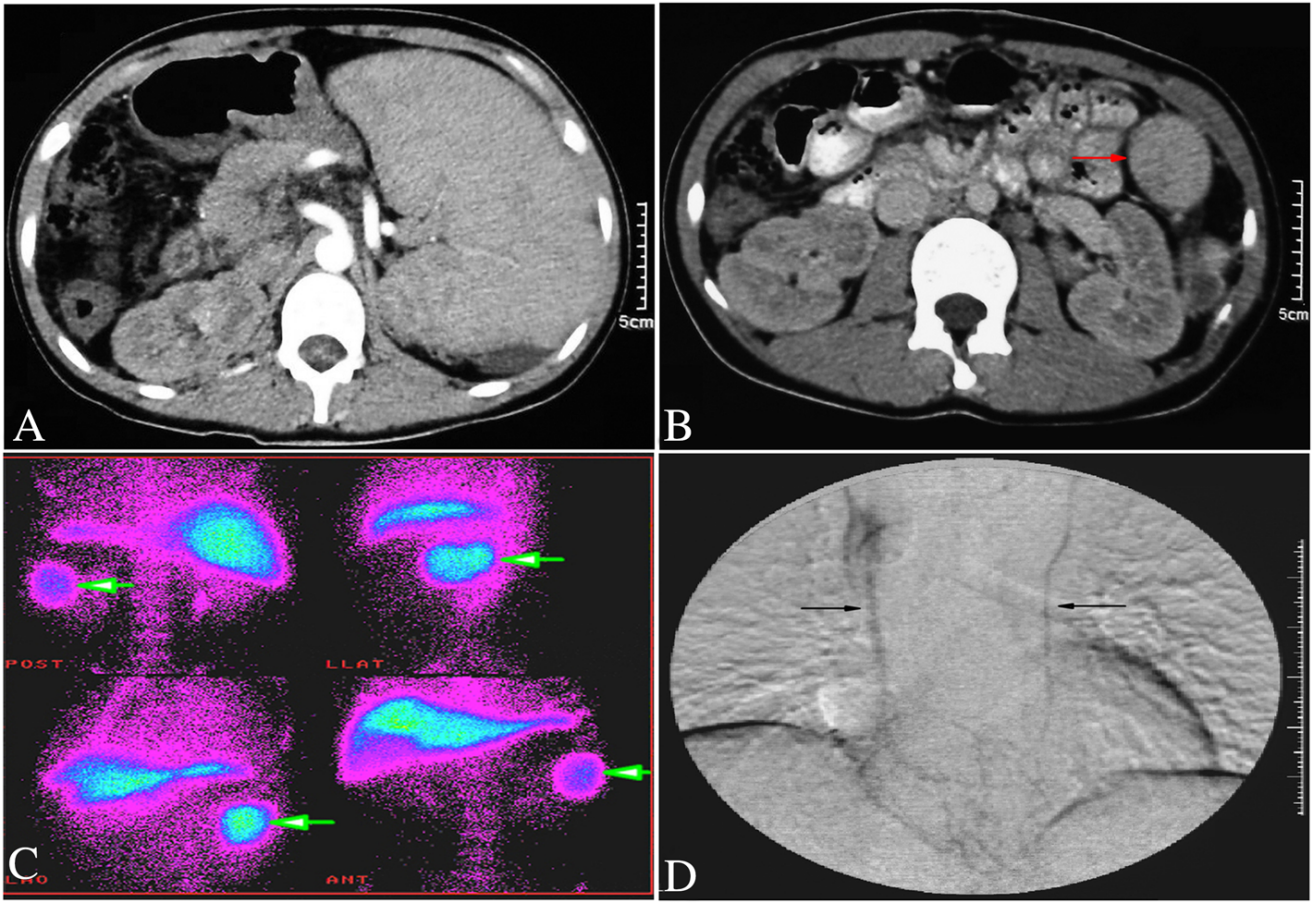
a CT scan revealed splenomegaly pre-operatively. b CT scan revealed that the blood supply of the residual spleen was normal (arrow) at 1-year, after SSFROM for PHT. c Isotope scanning with 99mTc showed that the residual splenic phagocytic function had recovered (arrow) at 4-years, after SSFROM for PHT. d DSA showed an established collateral circulation between the portal vein and superior vena cava (arrow) at 1 month, after SSFROM for PHT
